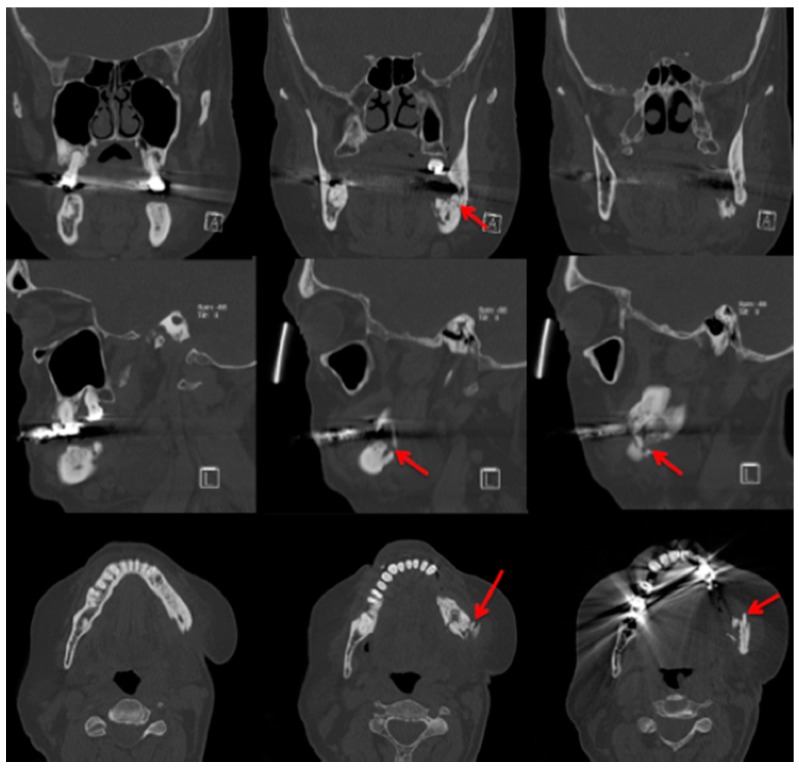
Computed tomography (Siemens, Sensation 64) Patient: 66 years old, male, secondary osteoporosis due to castration, alendronate. Red arrow points in the direction of the fracture due to the bisphosphonate necrosis. The panoramic radiograph is shown in Figure 3 and the 99Tcm-DPD SPECT and 99Tcm-DPD SPECT/CT in Figure 8 and Figure 9 respectively. AAMOS staging: stage 3.
